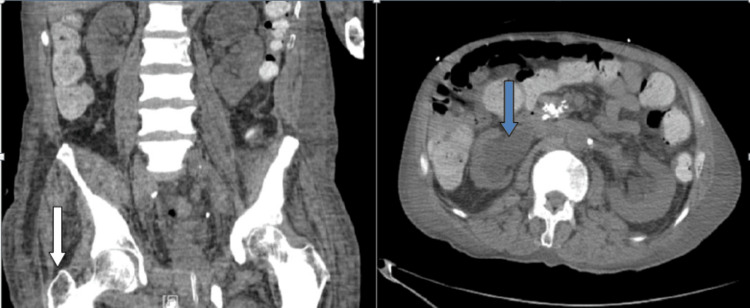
CT abdomen/pelvis showing severe patchy sclerotic changes (white arrow) with kidneys demonstrating hydro-nephrosis bilaterally particularly on the right (blue arrow)
